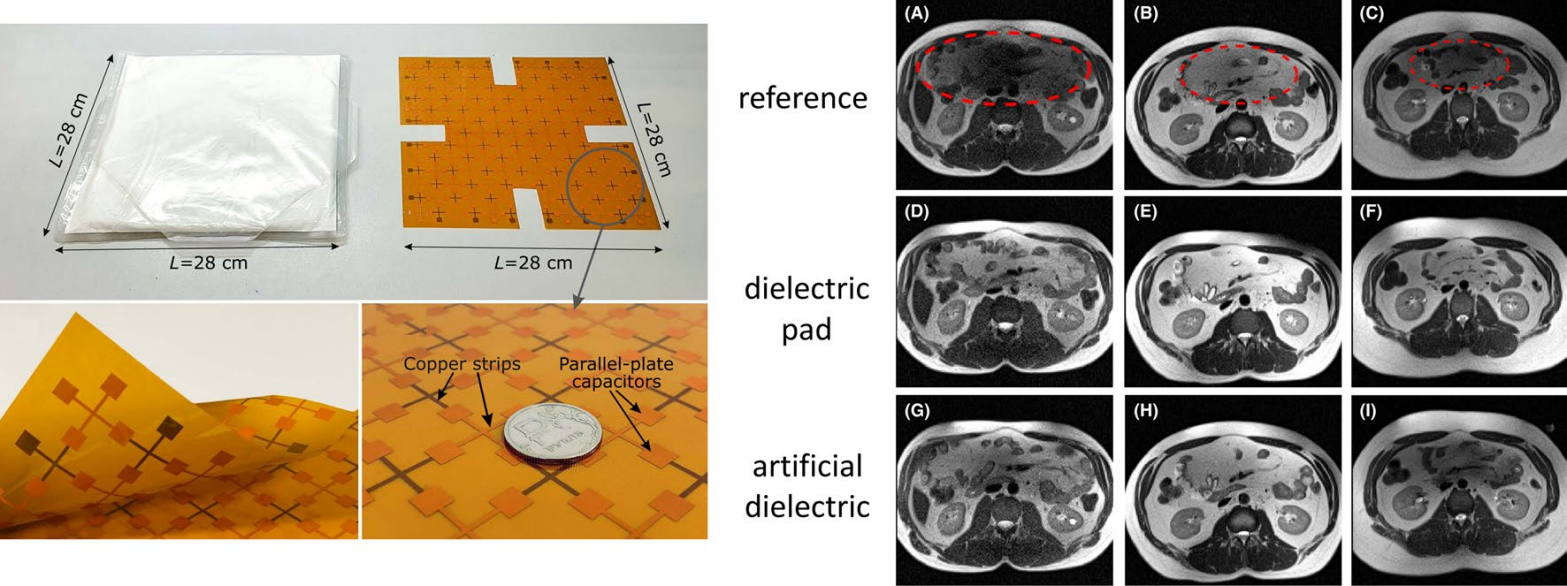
(Left) Photographs of a barium-titanate based dielectric pad (*ε*_r_ ~ 300) and an artificial dielectric/metasurface etched from a flexible double-sided PCB. (Right) 3 T MR images of three healthy volunteers. Figures reproduced with permission from Ref. [82]
